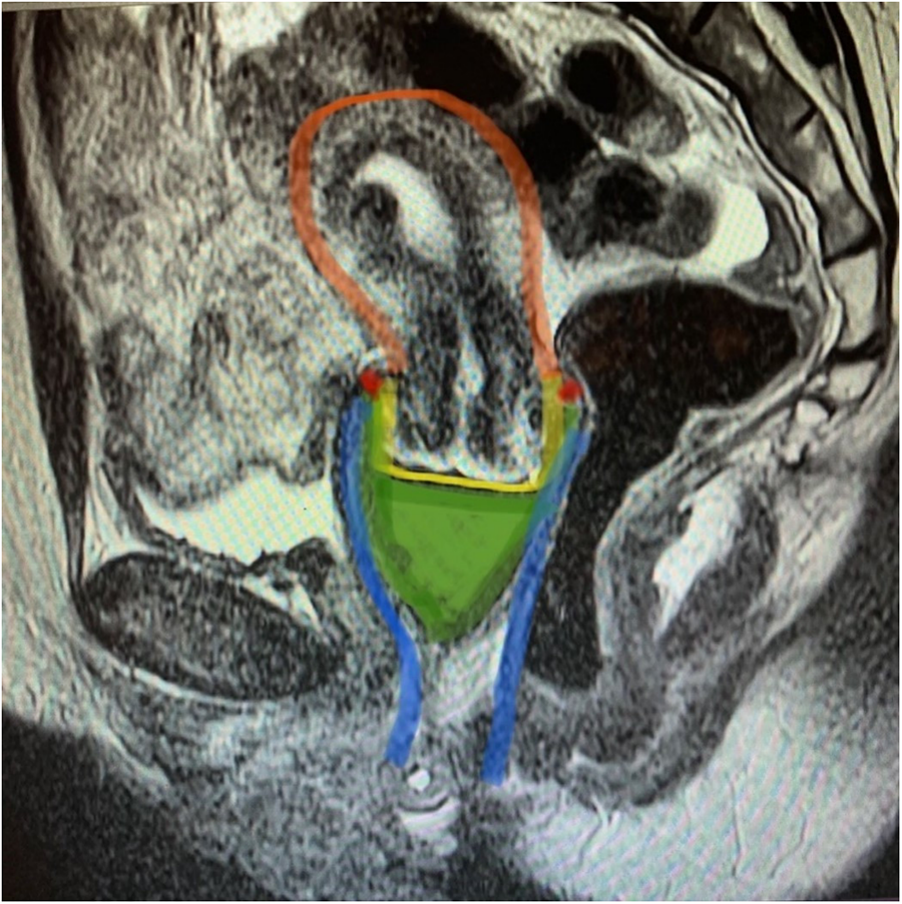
Magnetic resonance imagining of the Tampax menstrual cup in situ, sagittal view. MRI image T2 (transverse relaxation time) sagittal image of the menstrual cup in the female reproductive tract. Menstrual cup in situ contacts cervix and vaginal walls. Green: menstrual cup, blue: vagina, yellow: cervix, red: vaginal fornices, orange: uterus.
